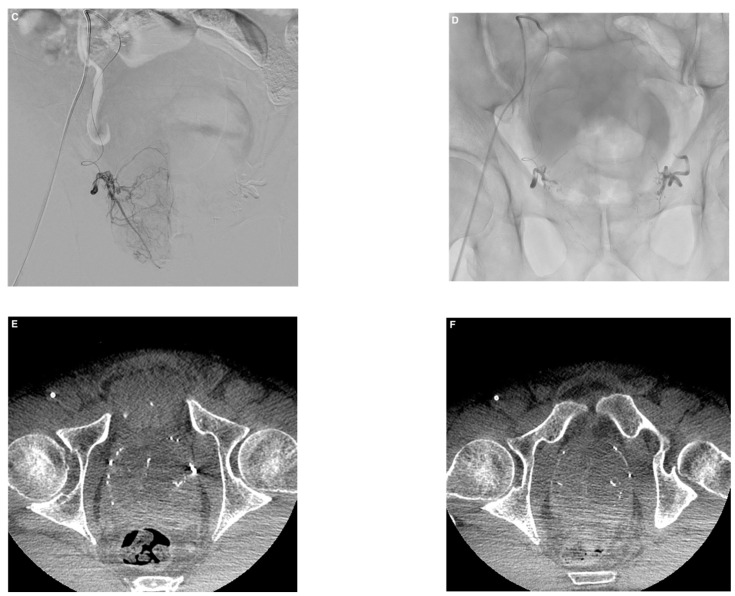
Example of prostate artery embolization (PAE) with N-butyl cyanoacrylate glue in a 74-year-old patient with symptomatic benign prostatic hyperplasia. (A) Left prostatic artery angiogram before PAE showing enhancement of the left prostatic lobe. (B) Follow-up angiogram after PAE with a mixture of Glubran^®^2/Lipiodol in a 1:8 ratio showing total occlusion. (C) Right prostatic artery angiogram with enhancement of the right prostatic lobe. (D) Visualization of the glue/lipiodol cast in the branches of the right prostatic artery after injection of the same mixture, with complete occlusion. (E,F) Axial cone-beam computed tomography images without contrast injection after bilateral PAE showing lipiodol uptake by both prostatic lobes with distal and proximal distribution of the glue/lipiodol casts. Major improvements in lower urinary tract symptoms were noted 3 months after PAE compared to baseline (IPSS, 5 versus 24; QoL score, 2 versus 6; prostate volume, 110 versus 170 mL).
